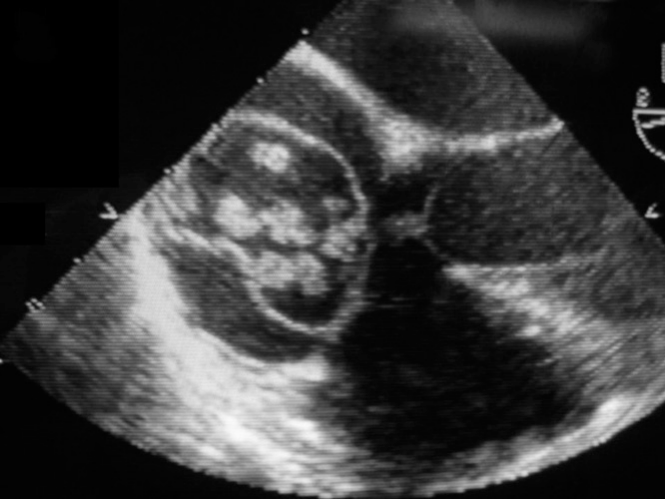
Initial manifestations of the patient with transesophageal echocardiography, showing a 4 × 3 cm cyst with multiple internal structures.
